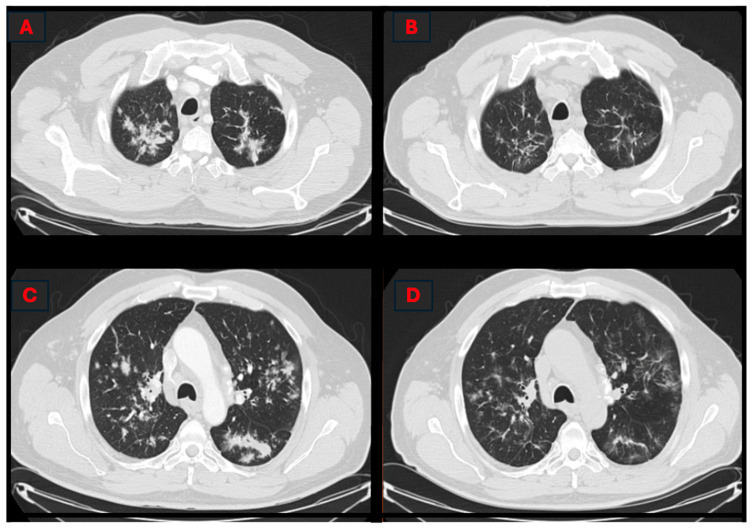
Axial CT images of the chest before (A,C) and after (B,D) treatment with immunosuppressive therapy, demonstrating marked reduction in parenchymal opacities and nodularity, consistent with radiologic improvement in pulmonary sarcoidosis.
